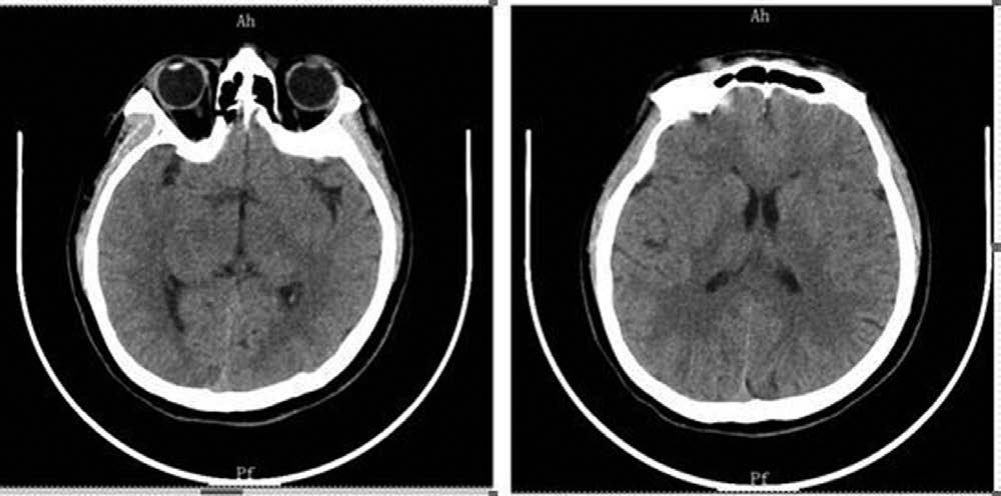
Head CT scan shows no abnormalities and seizures. The figure shows no identity of causes of seizure nor has any abnormalities. CT = computerized tomography.
